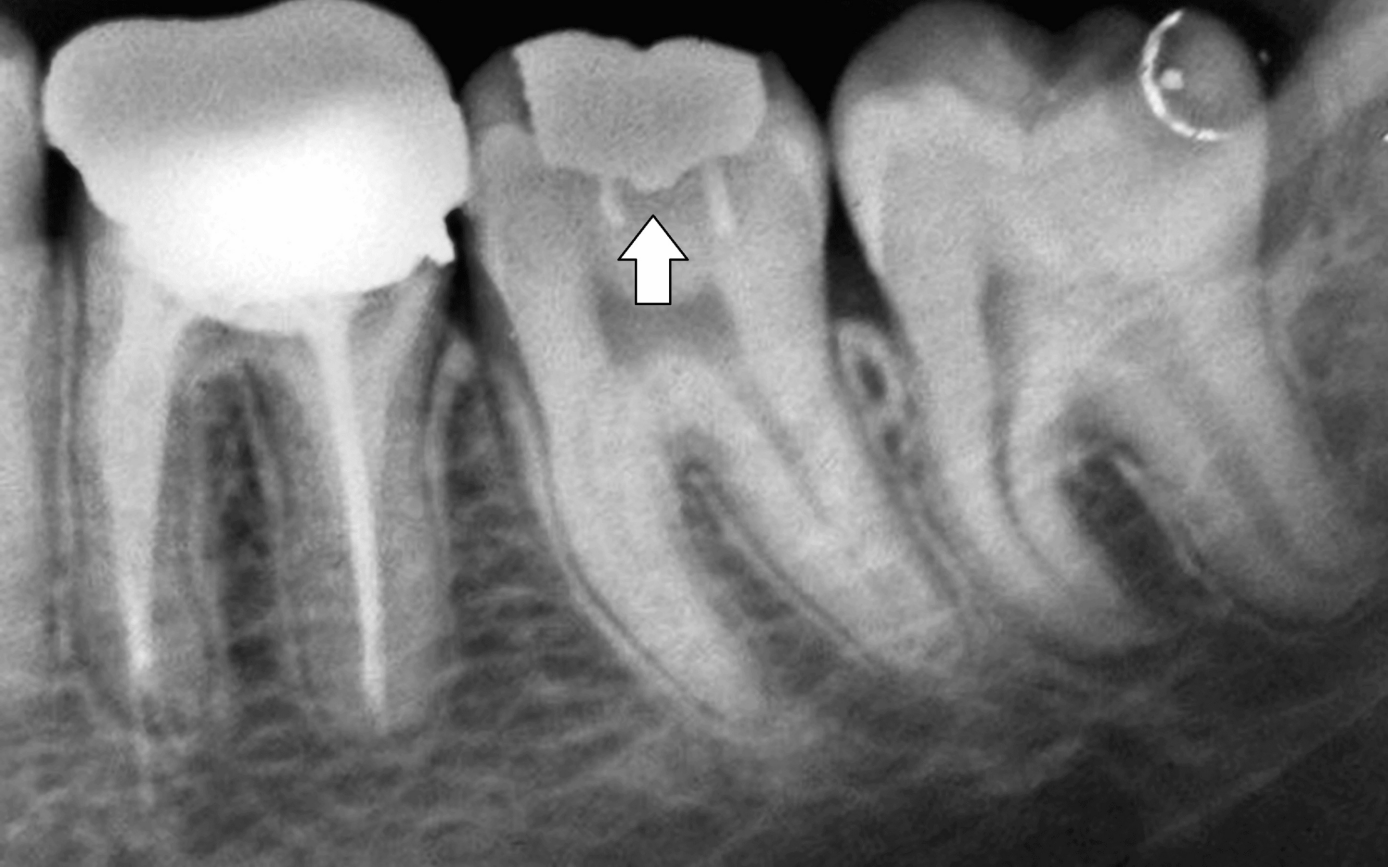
Radiographic presentation of pin-retained amalgam with respect to 37
